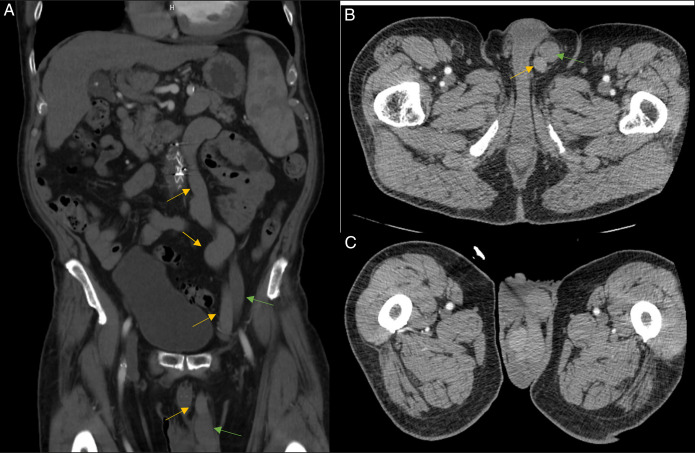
(A) Coronal view of abdominal/pelvic CT scan demonstrating dilated portal venous system vein (yellow arrows) and dilated central system vein (green arrows). (B) Axial view of abdominal/pelvic CT scan demonstrating portosystemic shunt. (C) Axial view of abdominal/pelvic CT scan showing dilated portal venous system (yellow arrow) and dilated vena cava system (green arrow). CT, computed tomography.
